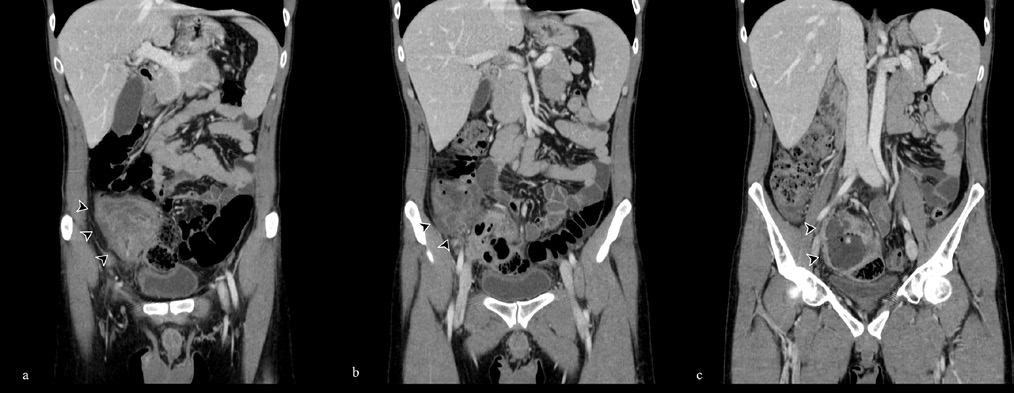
(a–c) Coronal post-contrast CT scan reconstructions in portal venous phase show the inhomogeneous abdominal-pelvic mass with discreet wall contrast enhancement (arrowheads).
